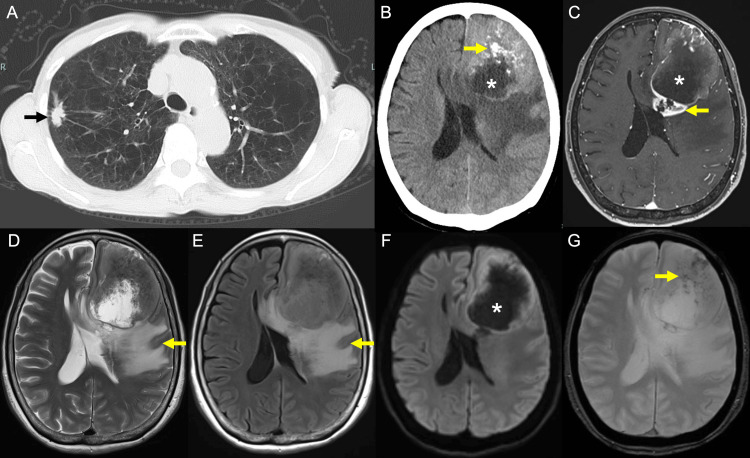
Preoperative CT and MR images (A) Chest CT demonstrates a solitary pulmonary nodule in the right upper lobe (arrow). (B) Non-contrast head CT shows a large, heterogeneous mass in the left frontal lobe measuring approximately 8 cm in diameter, with central coarse granular calcifications (arrow) and central hypodensity suggestive of necrosis (asterisk). (C) Brain MRI demonstrates a heterogeneously enhancing mass with peripheral ring enhancement (arrow) and a non-enhancing necrotic core (asterisk) on post-contrast T1-weighted imaging. (D, E) T2-weighted and fluid-attenuated inversion recovery (FLAIR) sequences demonstrate extensive perilesional hyperintensity, consistent with vasogenic edema (arrows in D and E). (F) Diffusion-weighted imaging (DWI) shows low signal intensity in the necrotic core (asterisk) without restricted diffusion at the enhancing rim. (G) T2*-weighted gradient-echo (GRE) sequences reveal intralesional signal voids suggestive of calcification and/or hemorrhage (arrow).
